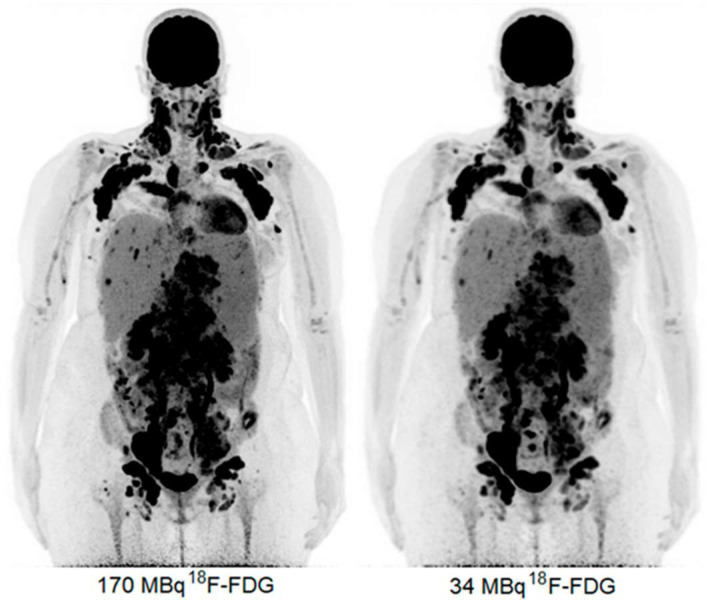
Maximum intensity projection (MIP) PET images acquired via a LAFOV Biograph Vision Quadra PET/CT scanner in a patient at 19 weeks of pregnancy with 170 MBq FDG (left) and reconstructed with 34 MBq FDG (right). Image (partially) taken from van Sluis et al. CC BY 4.0 License: http://creativecommons.org/licenses/by/4.0/ [39].
